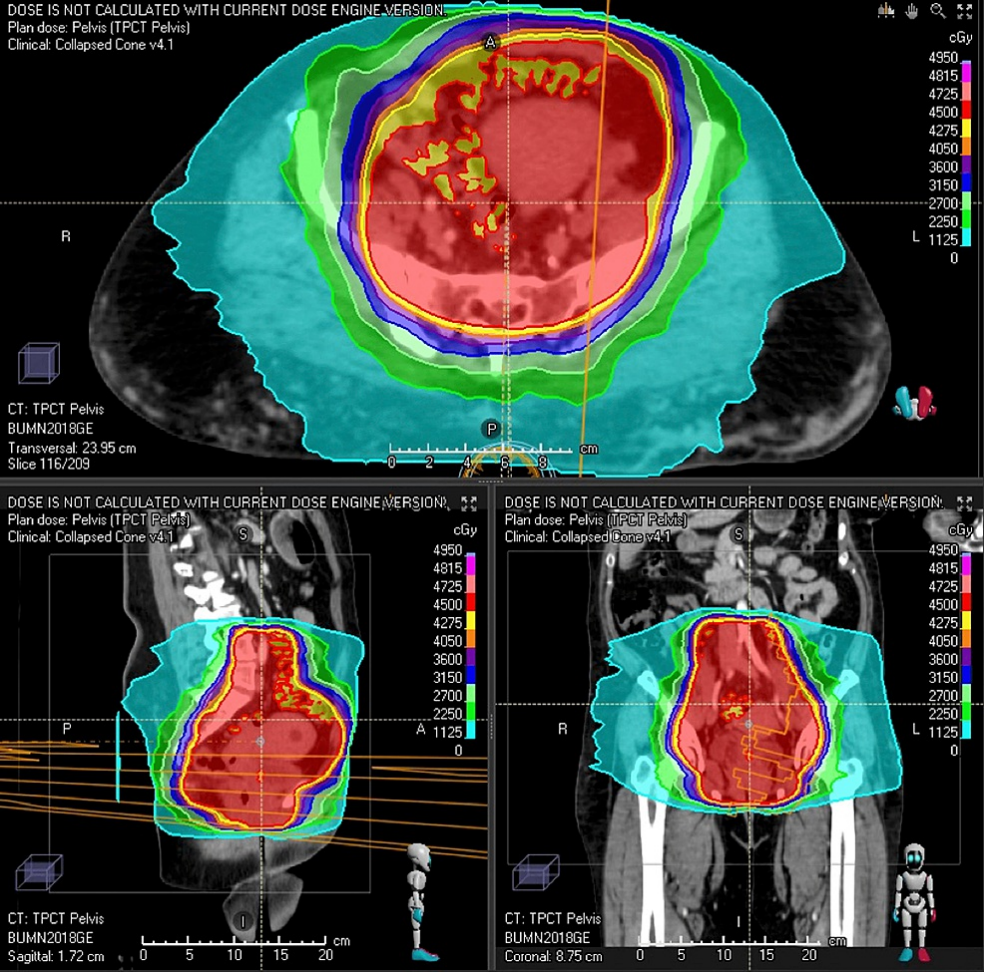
Radiation treatment plan External beam radiation treatment plan of the pelvis including lymph nodes and cervix/uterus/vagina which went to 45 Gy in 25 fractions. This plan was followed by brachytherapy to the cervix. TPCT: treatment planning computed tomography; cGy: centigray; A: anterior; P: posterior; R: right; L: left; S: superior; I: inferior.
